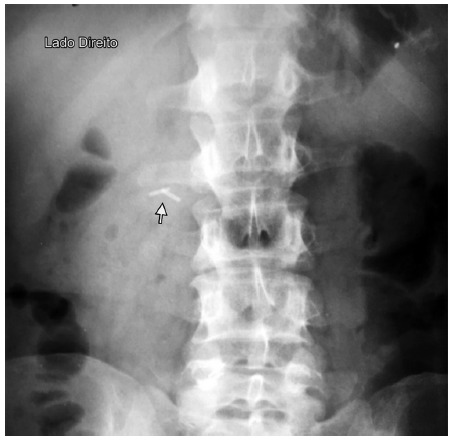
Simple abdominal radiographic study showing the two clips on the right side of the spine. In this case, the diagnosis of Petersen hernia was confirmed in laparoscopy
